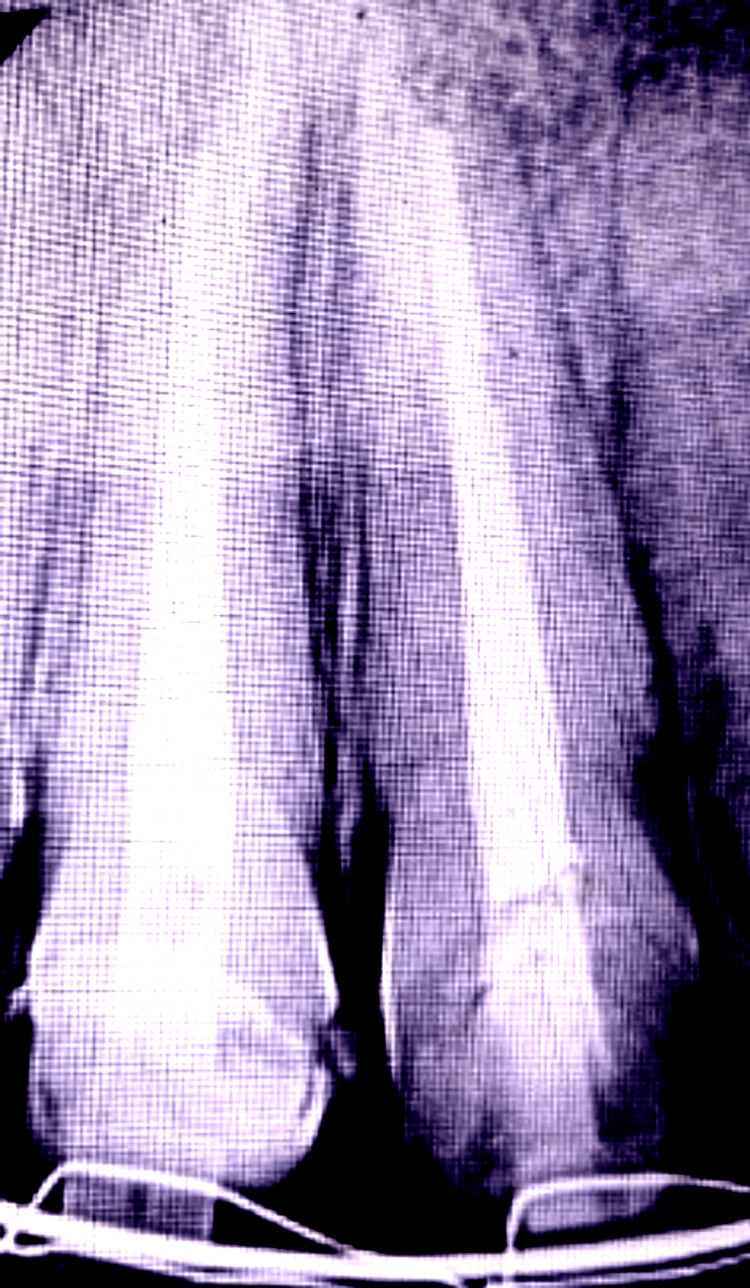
At T=1 year: Follow-up. Post-operative radiograph showing evidence of periapical healing in relation to tooth 21.
